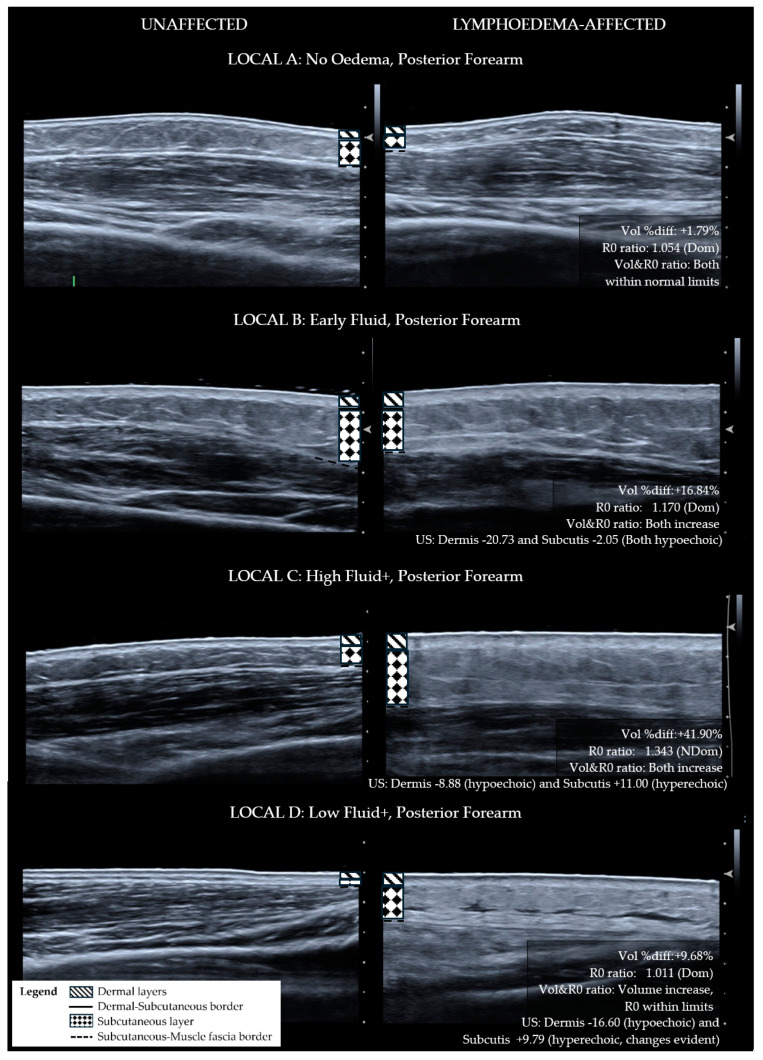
Ultrasound images for posterior forearm sites categorised with LOCAL criteria—matched lymphoedema-affected and unaffected sites. Lymphoedema-unaffected site (left), lymphoedema-affected test site (right). LOCAL A: No tissue characteristic change—the affected limb is also dominant which may explain lower subcutis and greater muscle thickness. LOCAL B: Lymphoedema dermal thickness increase and full-thickness hypo-echogenicity of the subcutis compared to the unaffected site. LOCAL C: Lymphoedema dermal thickness increase with hyperechoic change within the subcutis. LOCAL D: Lymphoedema dermal thickness increase with hyperechoic change along the dermal–subcutaneous border. Structural changes in fibrotic and fatty deposition have replaced normal tissue affecting the full thickness of the subcutis. Localised hypo-echogenicity within the subcutis is consistent with pools of fluid.
